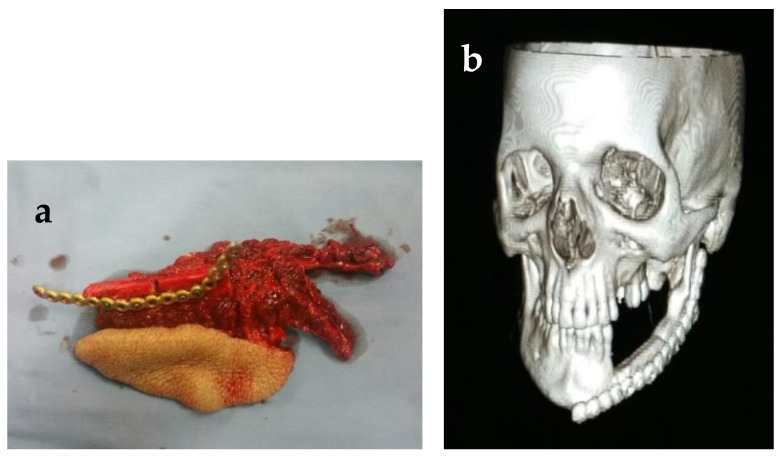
(a,b) Fibula graft, 3D computed tomography of patient showing hemimandibular defect reconstructed with fibula placed at the basal bone level.
